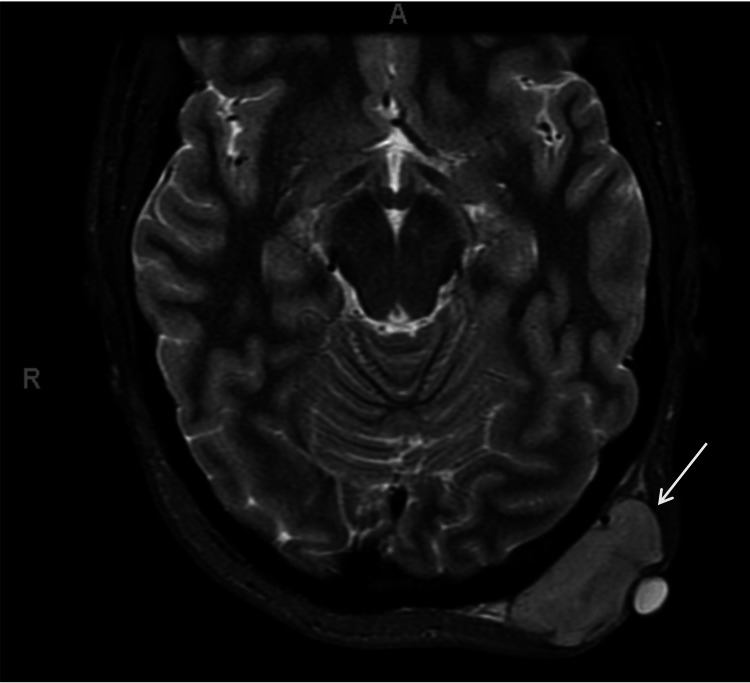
MRI demonstrating enhancing soft tissue mass without bony invasion (arrow) MRI: magnetic resonance imaging
